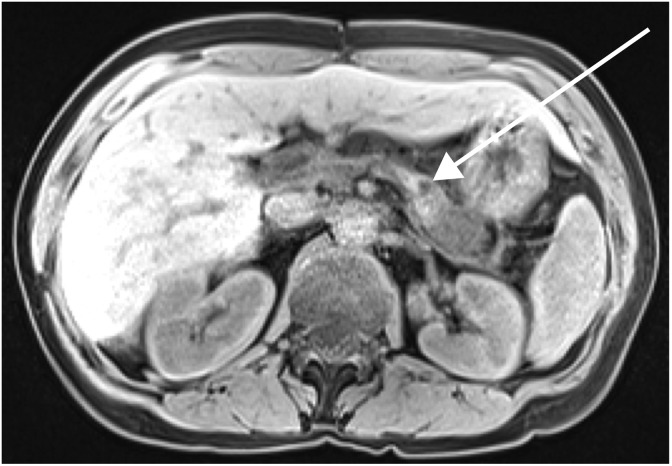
MRI (axial T1 weighted) of the 2017 pancreatic mass. The image was read to show interval development of a mass within the pancreatic body–tail junction with restricted diffusion and hypoenhancement. The mass was measured to be 2.3 × 2.2 cm (see arrow), and it was felt to focally encase the splenic artery and segmentally narrow the splenic vein.
